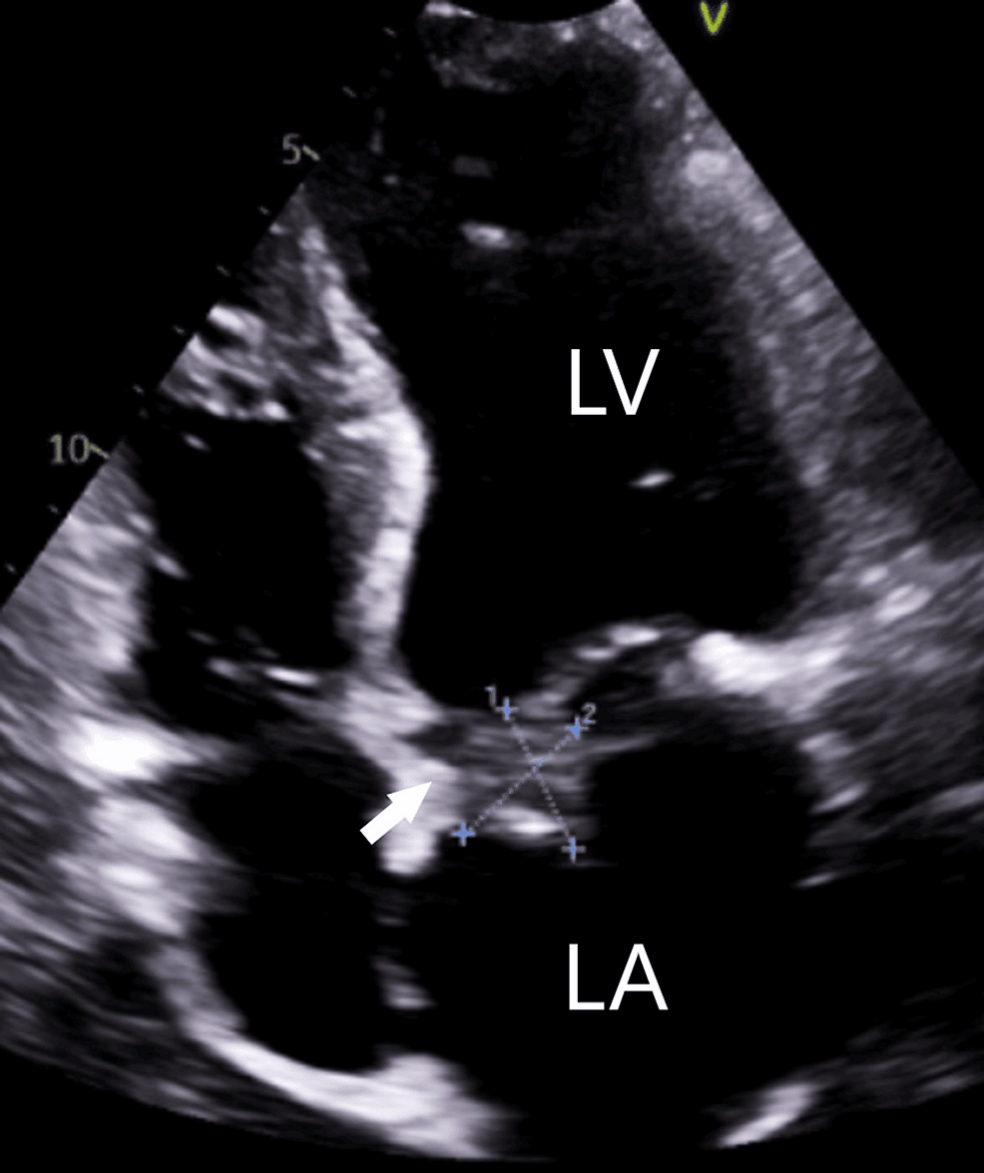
TTE showing a large 2.3 cm vegetation on the base of the posterior leaflet (arrow). LA, left atrium; LV, left ventricle; TTE, transthoracic echocardiogram
